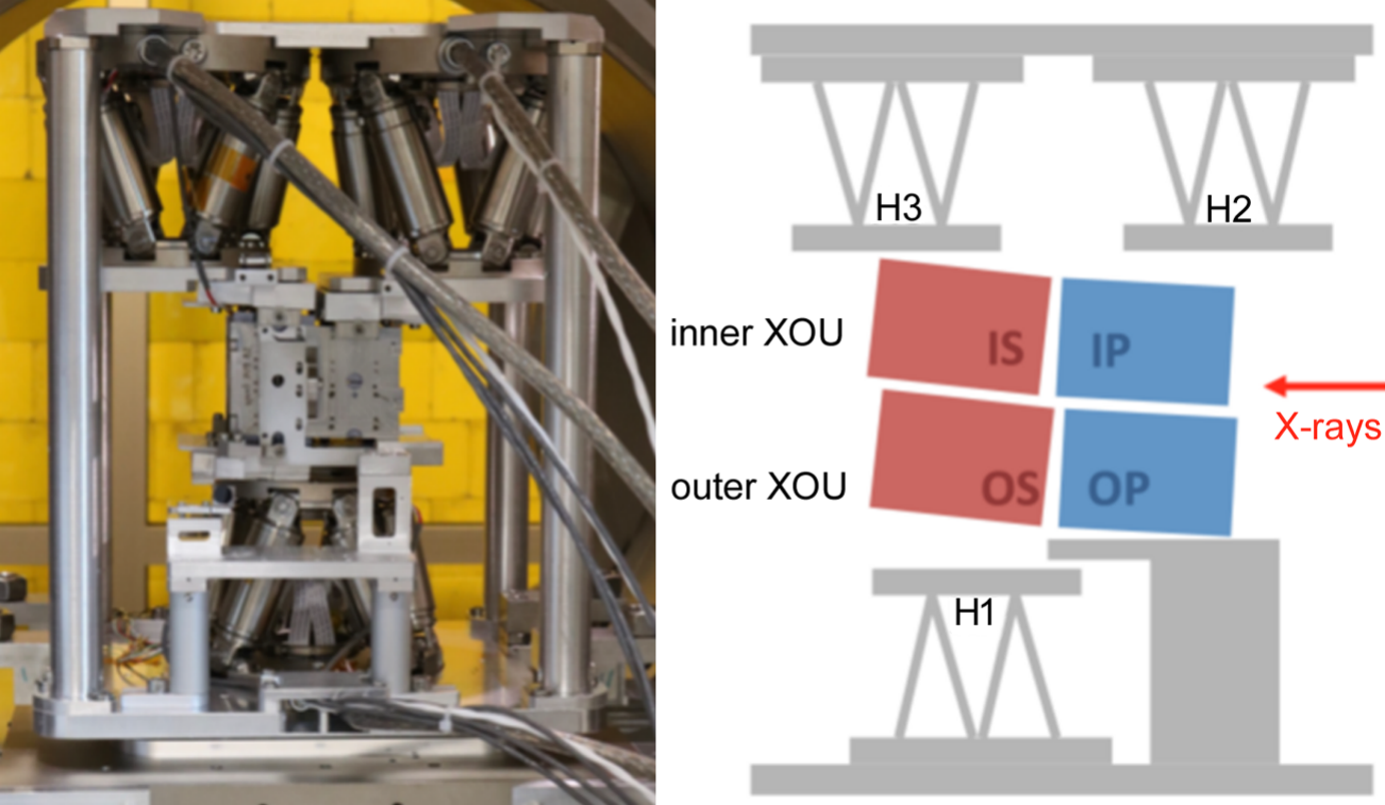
MM assembly at XPBF 2.0 using three small hexapods to align the mirror stacks: OS (outer secondary), IP (inner primary) and IS (inner secondary) with respect to OP (outer primary). For the alignment with X-rays, the setup is placed on the main hexapod in the vacuum sample chamber.
